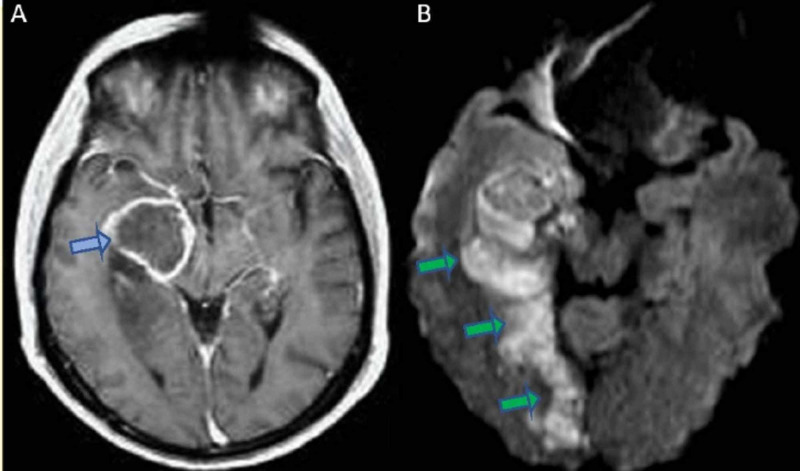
(A) MRI axial T1 gadolinium depicting a highly vascularized right temporal lobe tumor with an irregular ring enhancement (blue arrow). (B) DW-MRI scan showing a recent right posterior cerebral infarct in close proximity to the tumor (green arrows) DW-MRI: Diffusion-weighted magnetic resonance imaging
